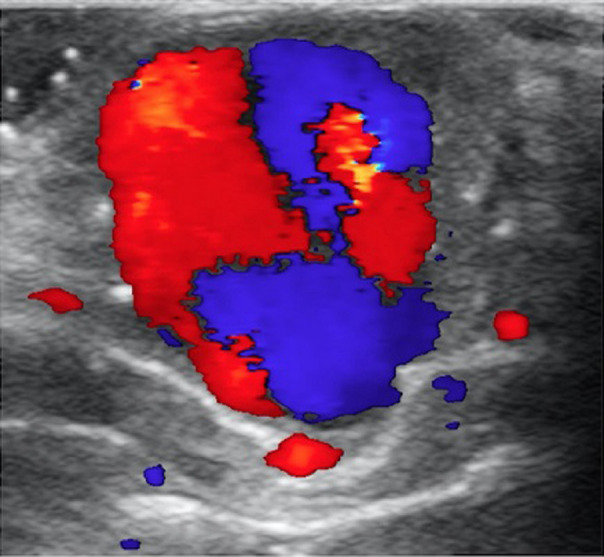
en mode doppler le caractère vasculaire circulant de la lumière est objectivé par le signe de Ying-Yang
